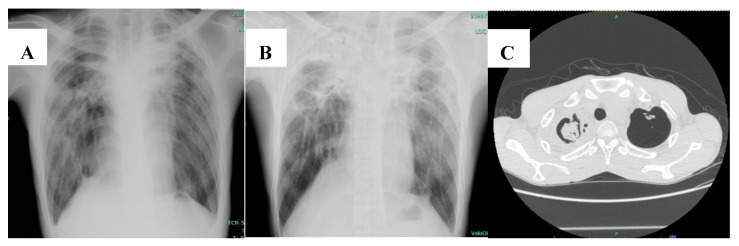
Chest imaging of a 37-year-old man diagnosed with chronic pulmonary aspergillosis following pulmonary *Mycobacterium avium* infection. (A) Chest radiograph showed cavitary lesion at right upper lobe (pulmonary *Mycobacterium avium* infection). (B) Chest radiograph 1 year later showed thickening of pre-existing cavities, with pericavitary infiltration (chronic pulmonary aspergillosis). (C) Computed tomography of the chest showed a fungal ball in the pre-existing cavity.
